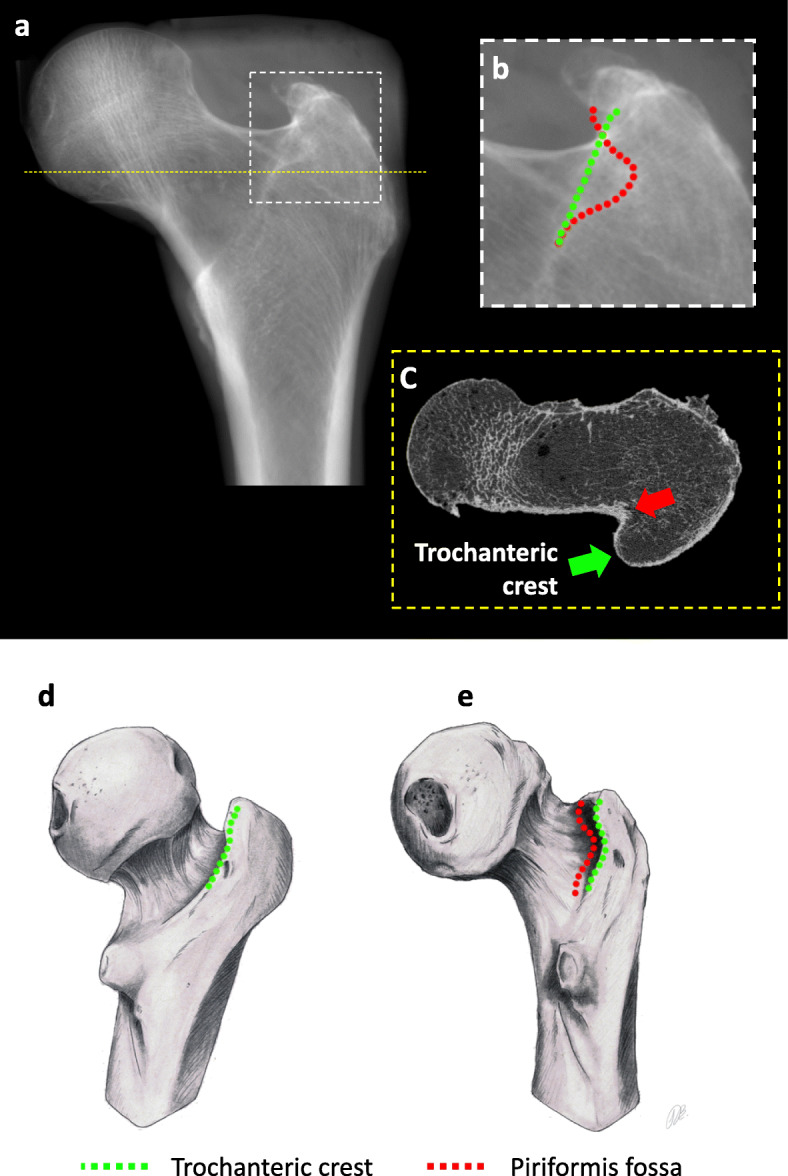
The double contour highlighted within the boxed area (a) on the pelvic AP radiograph comprises the most common aspect of one S-shaped line (outlined by red dots) and the second line with minimal curvature (green dots) (b). On a high-resolution CT scan (c), the origin of the contours are identified as the trochanteric crest (green arrow) and piriformis fossa (red arrow). In profile view, both lines are superimposed (d) and as the femur rotates, the two lines appear as separate elements (e)
